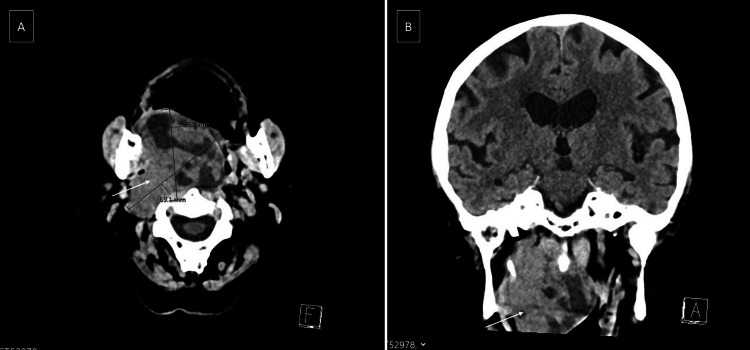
A) Axial CT showing a large, heterogeneous mass filling the entire oropharyngeal lumen (white arrow); B) same finding on coronal CT (white arrow).
